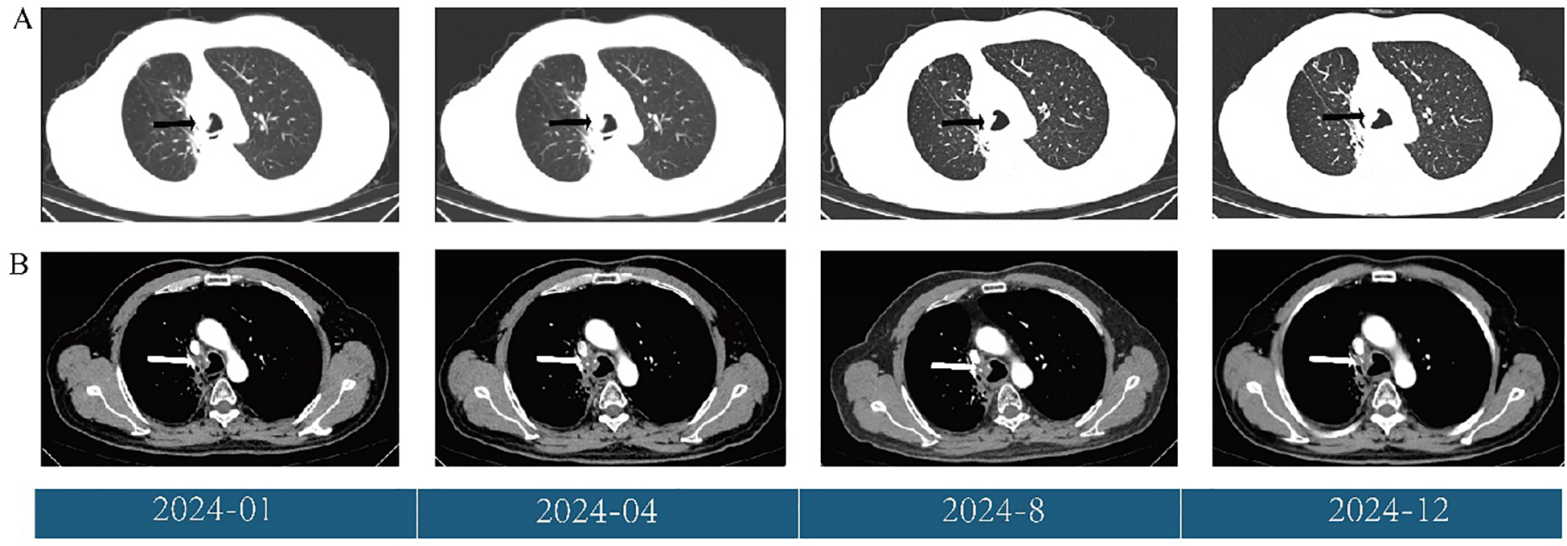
(A) Changes in the primary lung lesions (black arrow) during treatment, shown on CT (lung window). (B) Changes in the primary lung lesions (white arrow) during treatment, shown on CT (mediastinal window).
